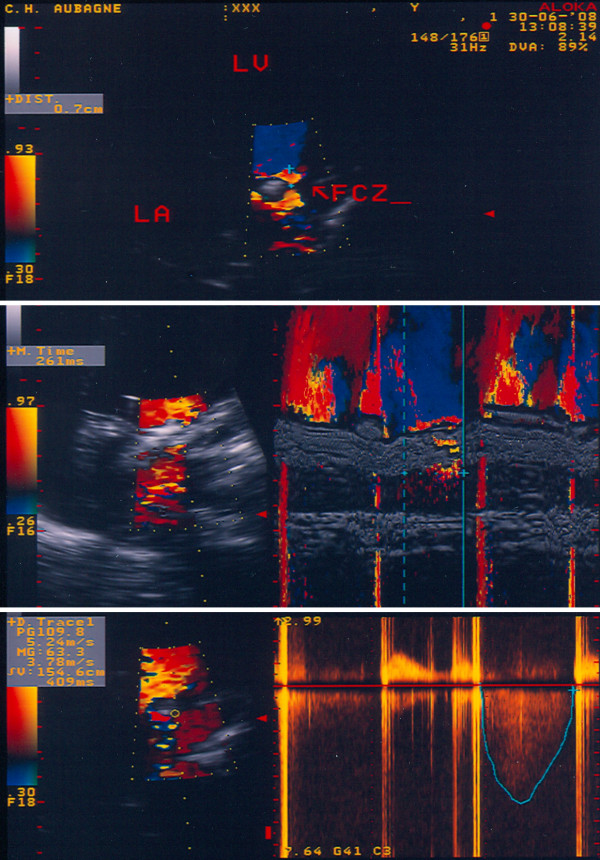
Upper part: measurement of the radius (r = 0.7 cm) of the flow convergence zone (FCZ, arrow) at mid-systole in a 4-chamber apical view, with an aliasing velocity of 30 cm/s. LA: left atrium; LV: left ventricle. Middle part: measurement of the regurgitation time (t, in secondes) by color M-mode at the level of flow convergence hemisphere; t = 0.261 secondes. Lower part: measurement of the velocity time integral (VTI, cm) and the peak regurgitant flow (Vmax, m/s) by continuous wave Doppler. VTI = 155 cm and Vmax = 5.2 m/s
